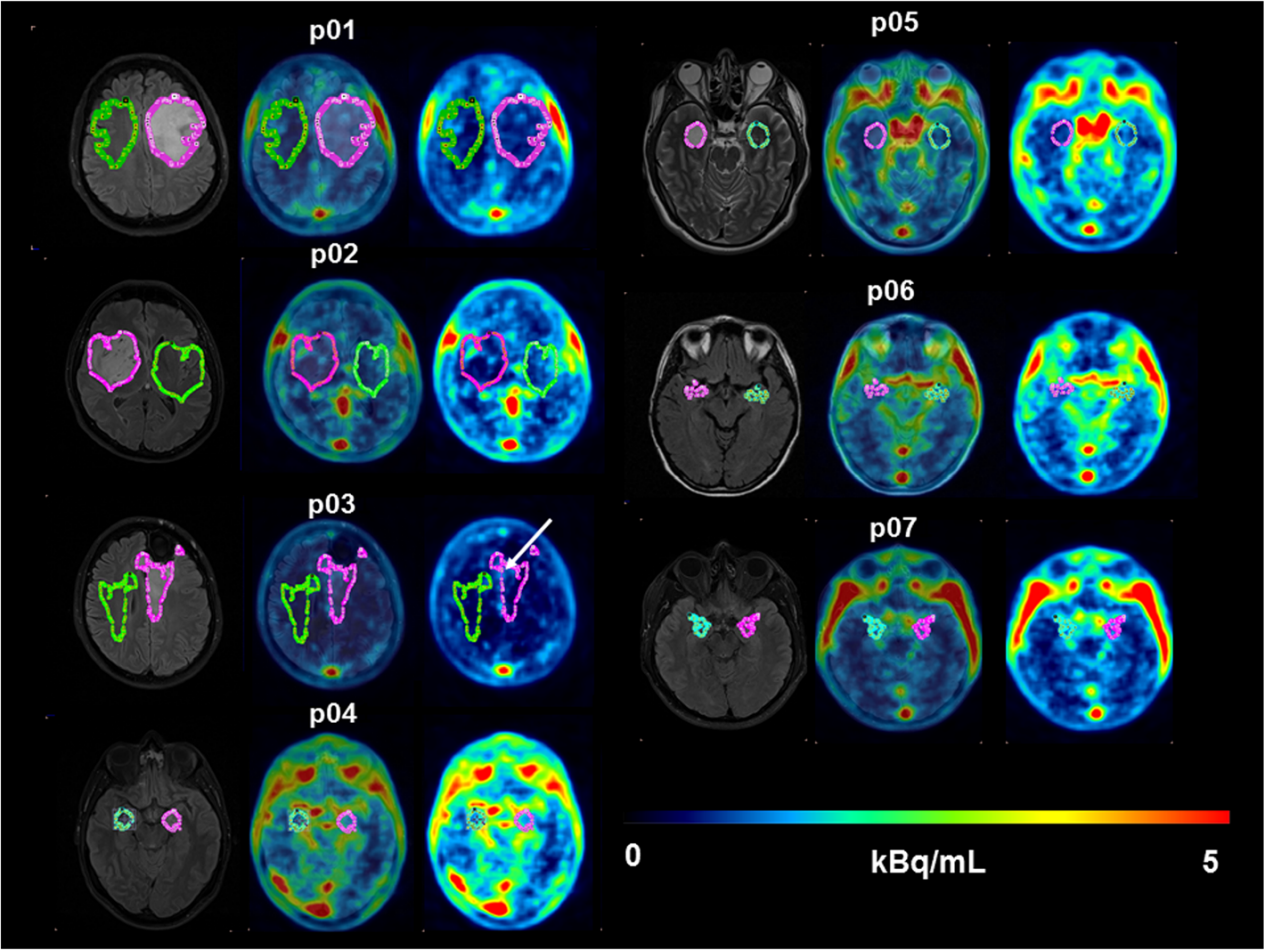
Transversal T2-weighted FLAIR MR images (left image), co-registered PET/T2-weighted FLAIR MR images (middle image) and [^11^C]tariquidar PET average images (0–60 min) (right image) in all patients. The contours for the tumor and contralateral tumor-free brain area are shown in pink and green colors, respectively. For p03, a tumor area with enhanced radioactivity uptake as compared with the rest of the tumor was visible (indicated by white arrow)
